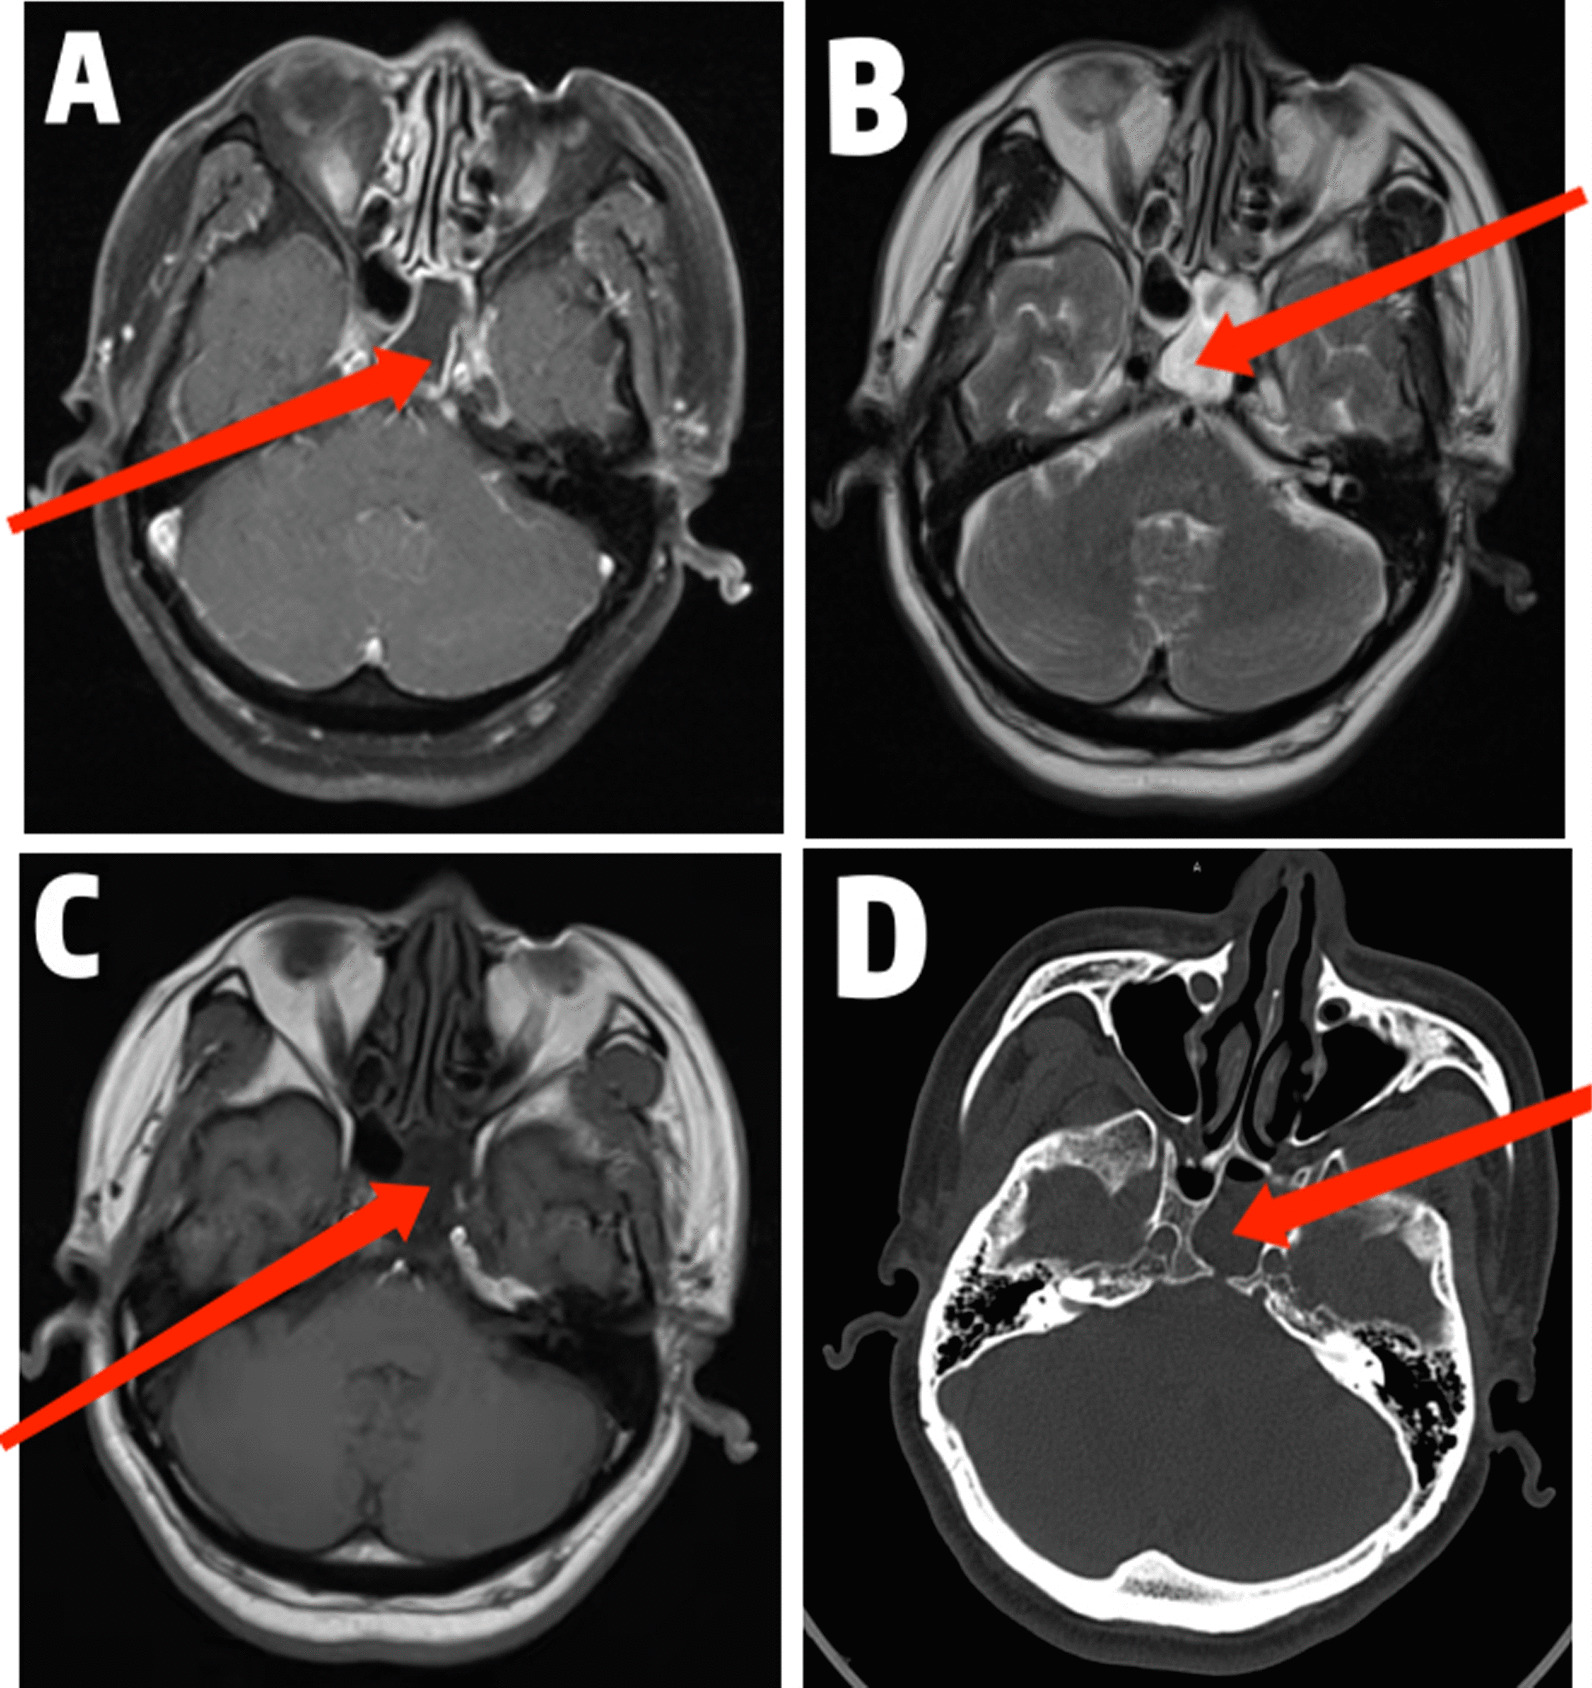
Imaging changes of the patient’s brain. MRI (A–C) and CT (D) indicates sphenoid sinus effusion as shown by the arrows
